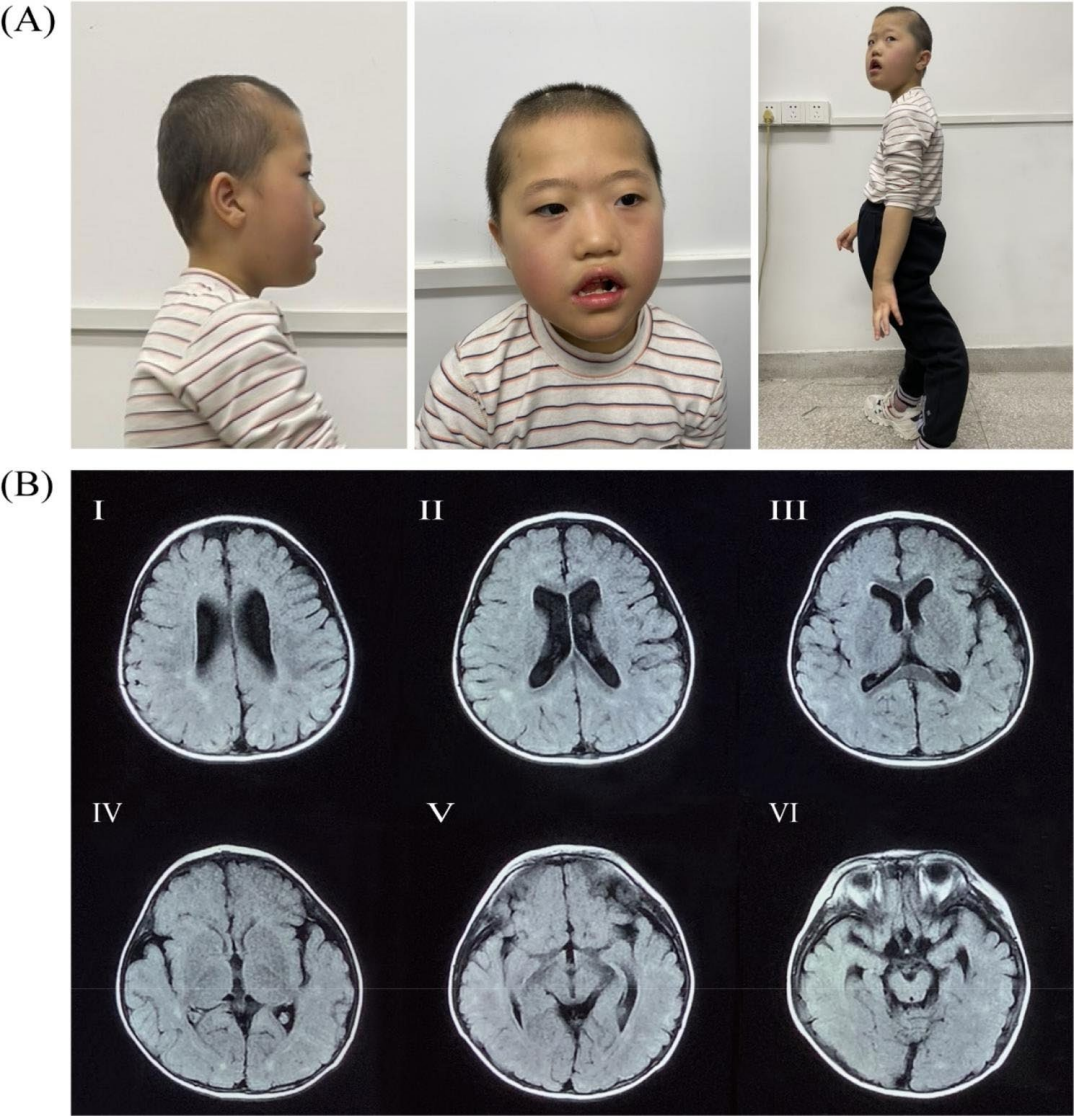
Facial appearance and brain MRI of the patient. (A) Typical KS1 facial features and hyperextended knees in our patient. (B) Axial T2 Flair images showing white matter hyperintensities
